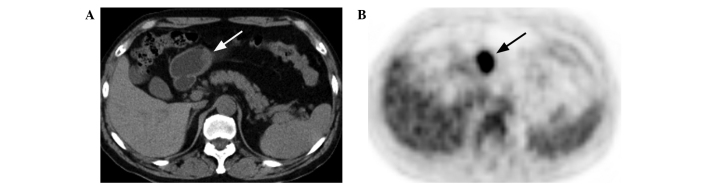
A 60-year-old male with gastric diffuse large B-cell lymphoma. (A) In the axial computed tomography image, a local thickened gastric wall can be observed in the antrum of the stomach. (B) In the axial positron emission tomography image, focal ^18^F-fluorodeoxyglucose uptake (maximal standard uptake value, 7) can be confirmed in the corresponding gastric region.
